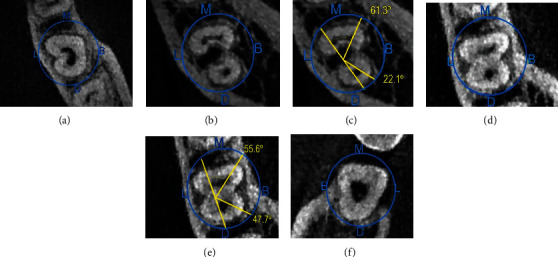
Different axial portions of CBCT images of mandibular second molars with C-shaped canals: C1 (a); C2 (b, c); C3 (d, e); C4 (f). (B: buccal; L: lingual; M: mesial; D: distal).
